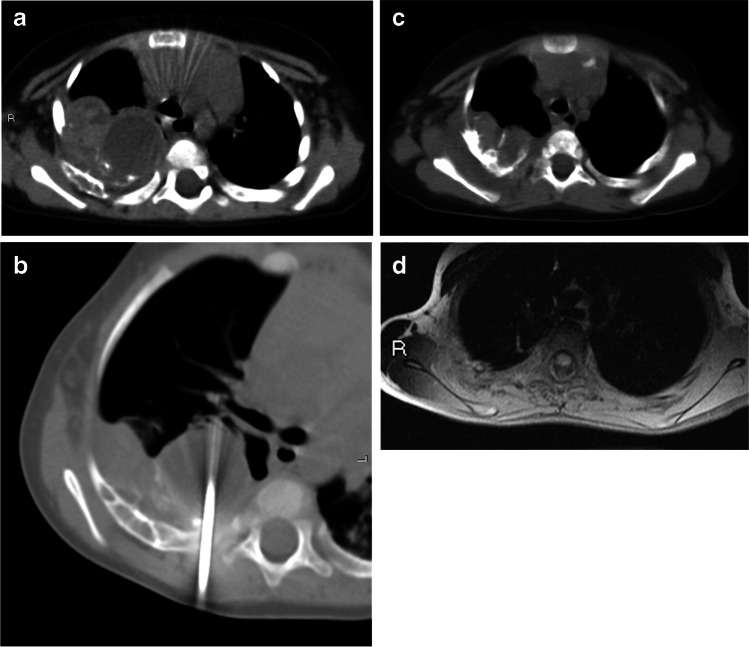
Mesenchymal hamartoma in a 6-month-old girl (patient 3) treated with radiofrequency thermoablation. She presented with persistent respiratory distress requiring diagnostic studies including chest radiograph and, successively, CT scan. a Axial CT shows a inhomogeneous oval mass arising from the 1st–2nd right ribs. b Two sessions of radiofrequency thermoablation were performed (axial CT). c, d Six-month follow-up images show a significant dimensional decrease of the tumor, as a smaller inhomogeneous mass with calcifications at axial CT (c) and as hypo/isointense residual disease on axial T1-weighted MRI (d)
